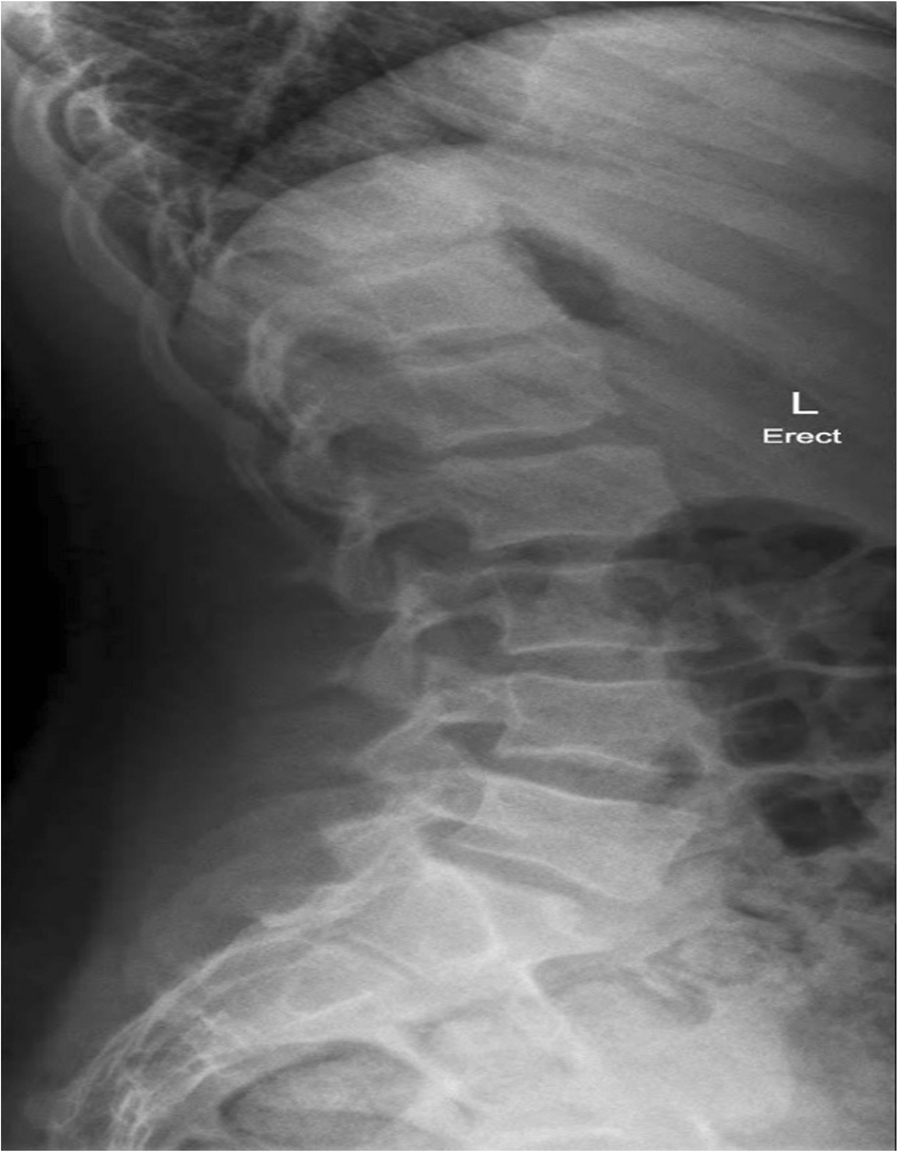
Lateral lumbar X-ray view. Note the suspicious area of increased sclerosis in the anterior half of the sacral base.
